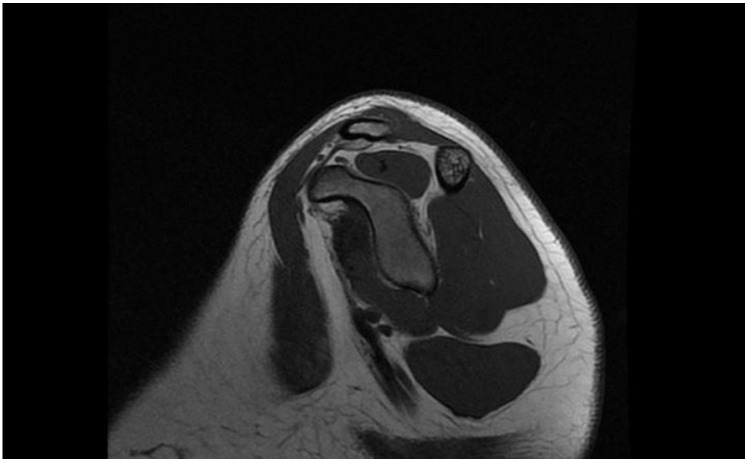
Trapezoidal ligament integrity in preoperative magnetic resonance imaging (MRI).
